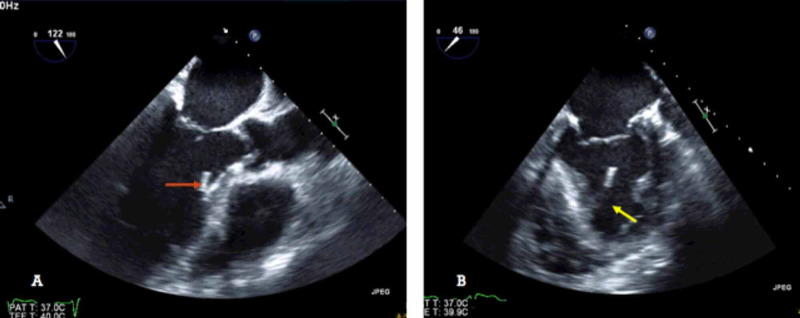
Transesophageal echocardiogram A: showing a smooth, sharp, linear echodensity (red arrow) not consistent with vegetation. B: an off-axis image showing the echodensity, part of the false tendon (yellow arrow).
